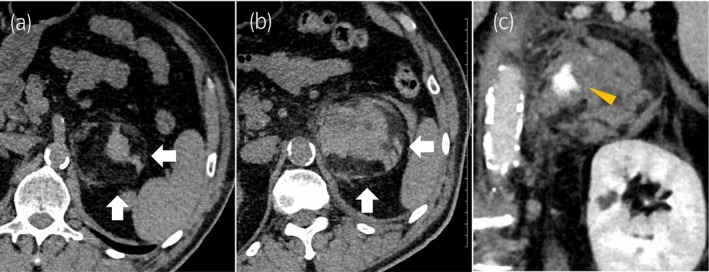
Computed tomography radiographs of the patient. (a) Abdominal CT scan showing a left AML of 68 × 58 mm^2^ before spontaneous hemorrhage (white arrow). (b) Abdominal CT scan showing a left AML with spontaneous hemorrhage of 89 × 83 mm^2^ (white arrow). (c) Abdominal CT with contrast enhancement (coronal image) demonstrated a focus of extravasation (yellow arrowhead).
